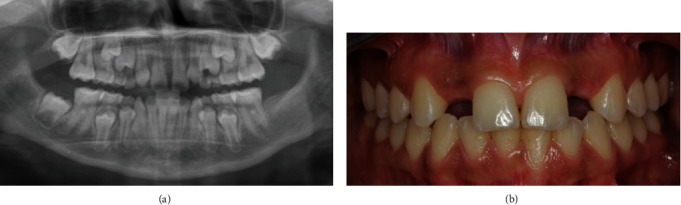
(a) Panoramic radiograph showing agenesis of both maxillary lateral incisors. (b) Facial view at the end of the orthodontic treatment showing the space maintained with the removable orthodontic retainer.
